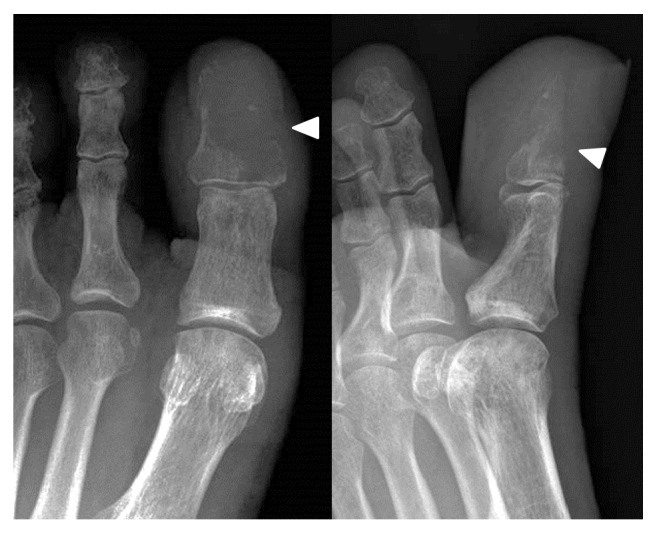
Plain radiographs of the left foot show destruction of the first distal phalanx.
